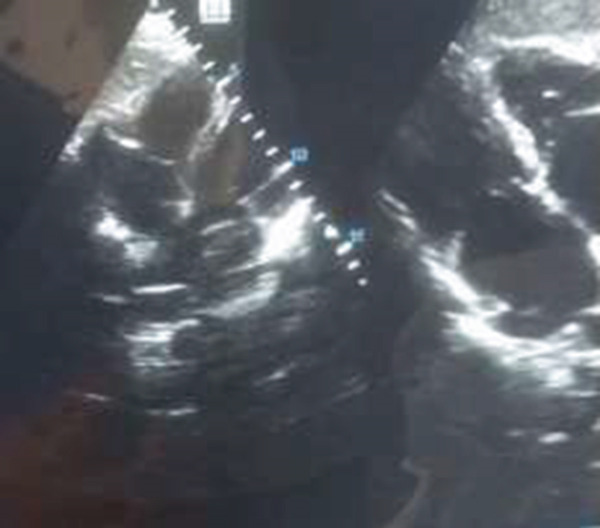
Transthoracic echocardiography of the patient in subcostal four‐chamber view showing normal cardiac chambers with apex directed towards the right of the patient′s chest.
